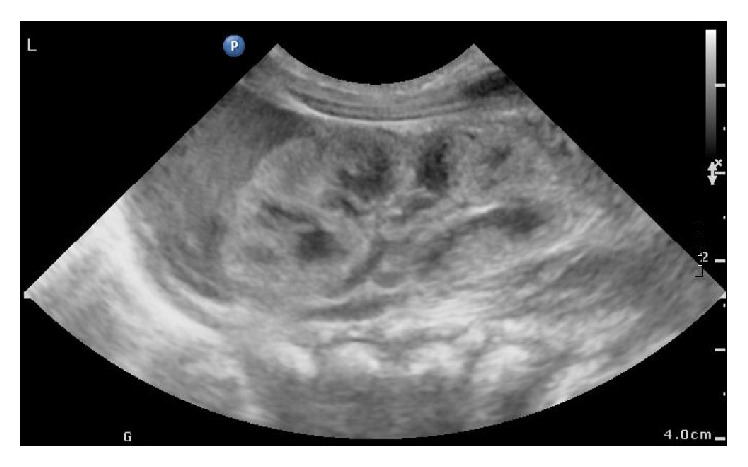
Normal looking left kidney in abdominal ultrasonography.
